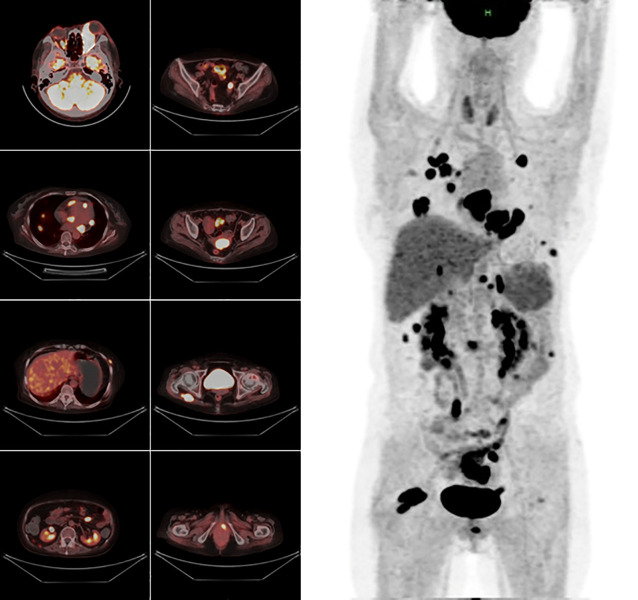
Whole-body PET/CT Scan. Hypermetabolism was noted in numerous muscles, lymph nodes, nodules, and visceral organs, indicating lymphoma with multisystem infiltration.
